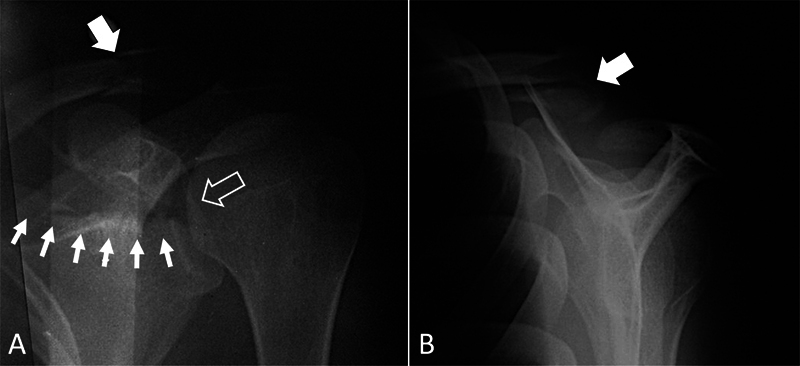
Radiographs of the left shoulder. The anteroposterior view (A) demonstrates the transverse and displaced scapular fracture involving the glenoid cavity and extending to the base of the coracoid process, classified as Ideberg type III (small arrows); detail of the joint displacement (large empty arrow); detail of the fracture in the distal clavicle (large solid arrow). The lateral view (B) details the displacement in the distal clavicular fracture (large solid arrow). Source: authors' archive.
